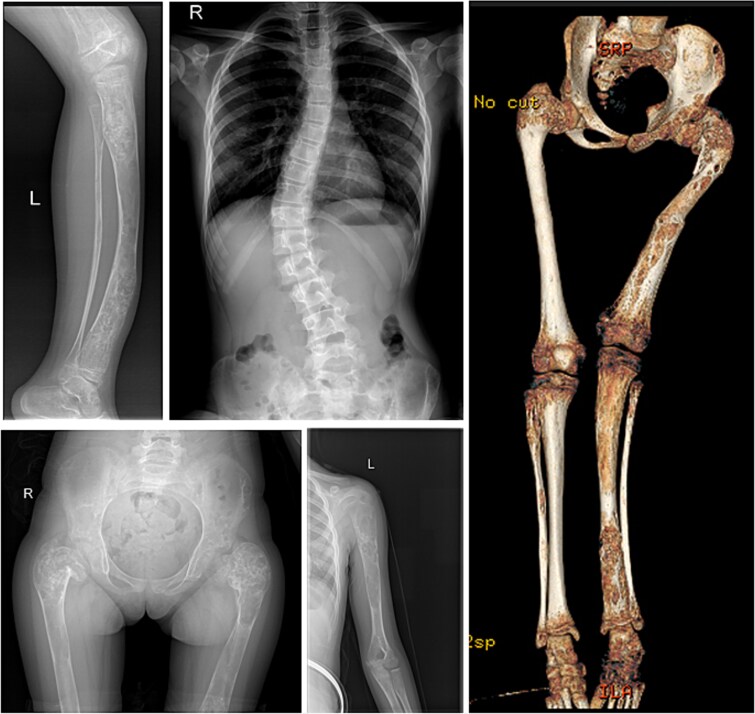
X-rays and 3D CT image of the patient showing the severity of the disease.
